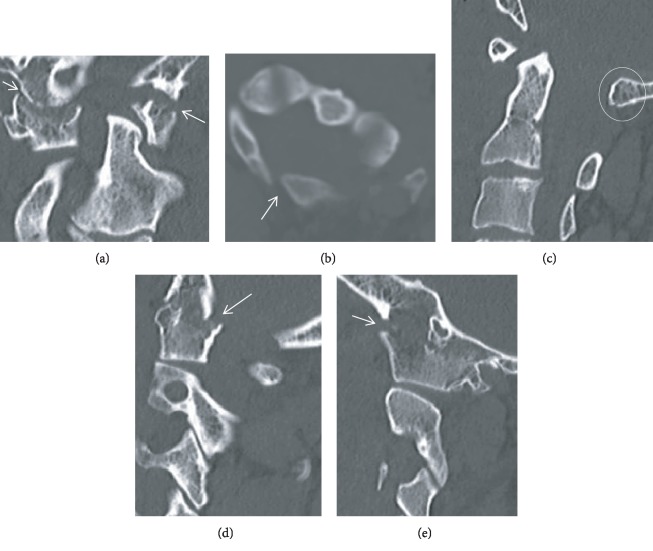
Case 2: cervical CT. C1 congenital partial assimilation to the skull base. (a) The right C1 lateral mass is partially fused with the right occipital condyle. The left C1 lateral mass is completely fused to the left condyle. The tip of the dens is dysplastic and asymmetrically positioned towards the left lateral mass. (b) The rachischisis of the C1 posterior arch. (d, e) Acute fracture dislocation of the previously right and left lateral mass assimilation, respectively.
